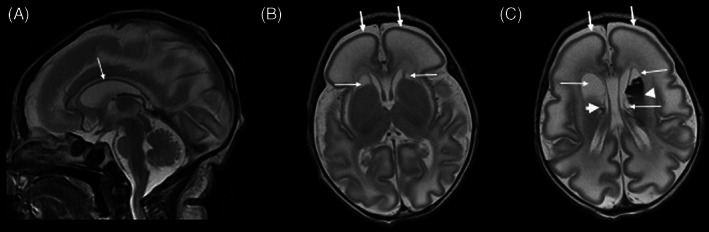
Brain magnetic resonance imaging in our patient day 6 day of life (corrected gestational age 35^+3^weeks). A, Sagittal T2 weighted image demonstrates small cerebrum and cerebellum with very thin corpus callosum. B and C, Axial T2 weighted images demonstrate simplified gyral pattern with thin lissencephalic cortex more severe in the frontal lobes (thick arrows), decreased white matter volume with markedly abnormal T2 hyperintensity, bilateral periventricular cysts (thin arrows) with blood products in the large left sided cyst and in a tiny right sided cyst (arrowheads). Prominent ventricles and large extra‐axial CSF spaces in keeping with small brain
